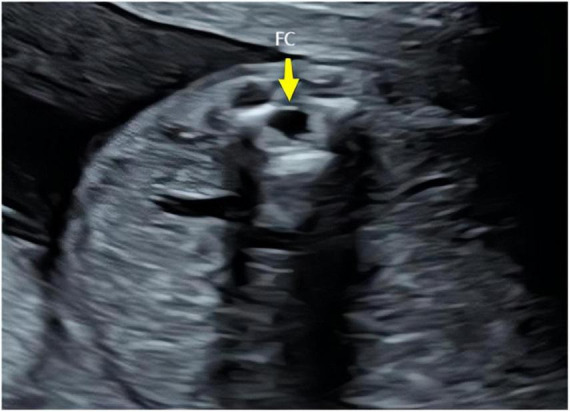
Transverse sections of the prenatal ultrasound of the spine. FC, terminal filament.
